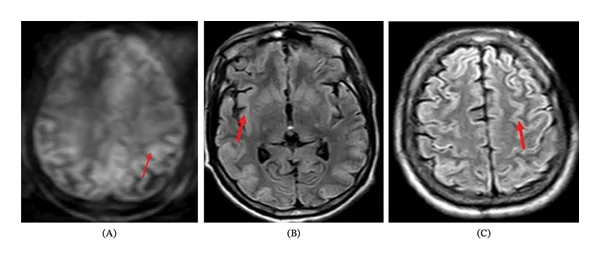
Brain MRI (A) Axial T2WI, (B) Axial T2WI‐FLAIR, and (C) Axial DWI pulse sequences show bilateral predominantly subcortical cerebral and cerebellar hemispheres, bilateral basal ganglia (caudate nucleus, lentiform nucleus, and thalami), and hippocampi with hyperintense signal changes and diffusion restriction in the DWI image (red arrow).
